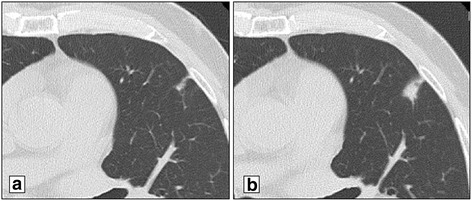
Imaging studies of the third pulmonary metastasis. Follow-up chest CT (98 months after curative resection of the primary pancreatic tumor) shows a newly appearing nodule in the upper lobe of the left lung (a). Chest CT (108 months after curative resection of the primary pancreatic tumor) shows that this nodule has increased (b) and appears to be a pulmonary metastasis from PDAC
